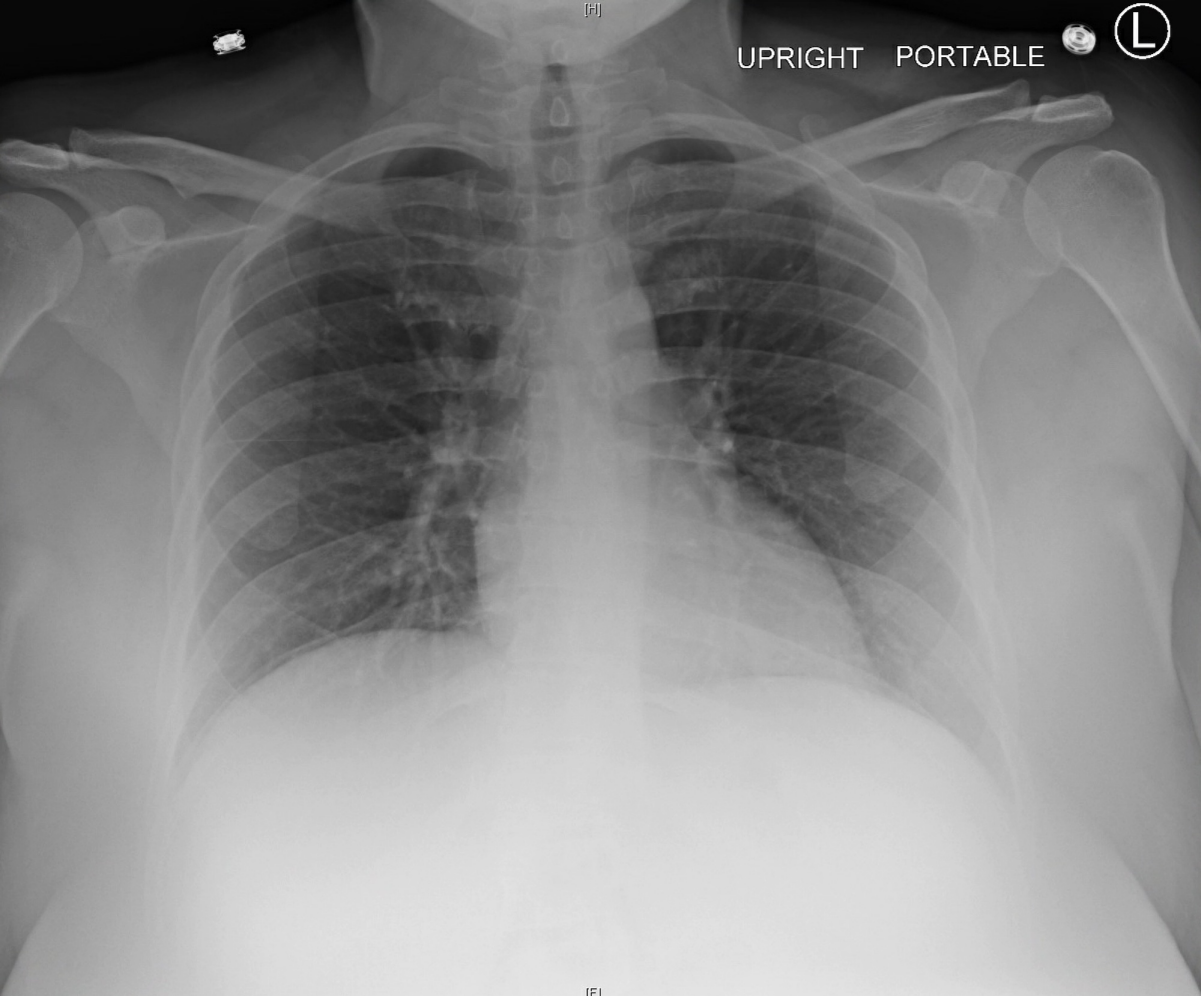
Chest X-ray of the patient No acute pulmonary disease noted L: left; [H]: head; [F]: foot
